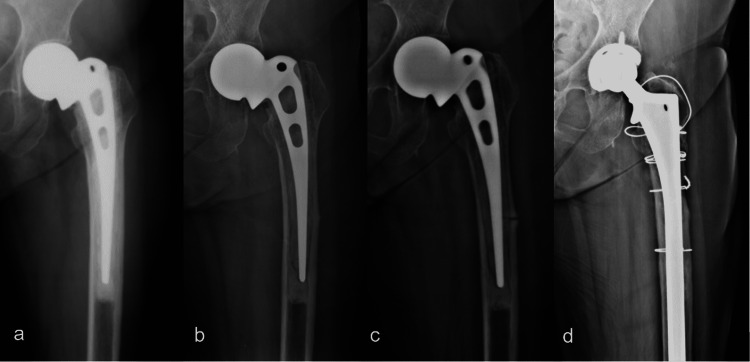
The radiographs from Case 1 (a) Post-operative hemiarthroplasty before APFF occurred, (b) focal lateral cortical thickening with clinical thigh pain at presentation, (c) visible radiolucency line at the lateral cortex thickening, (d) healing of the fracture at eight months after post-operative revision total hip arthroplasty with long femoral stem.
